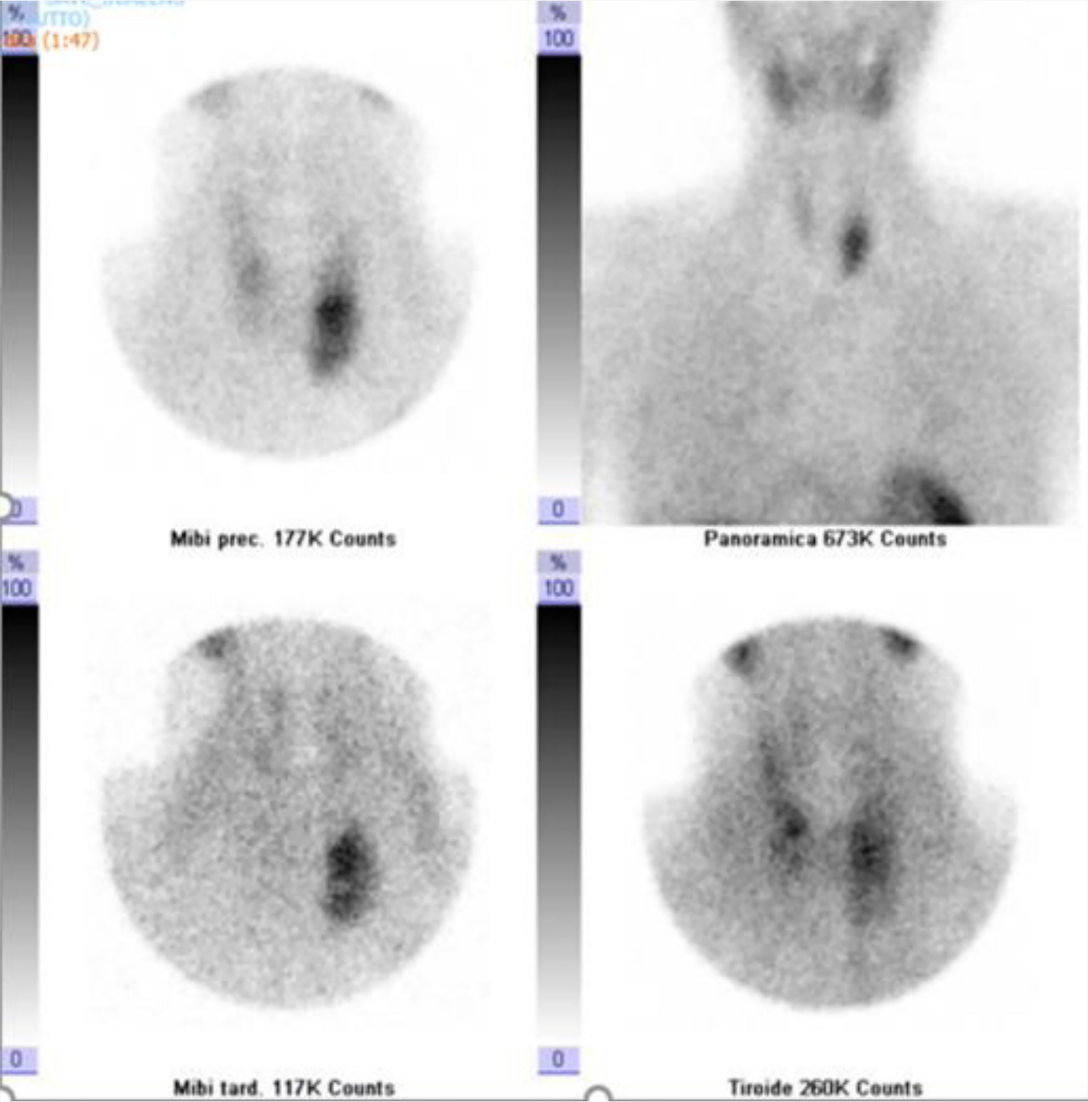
Dual-phase Tc-99m sestamibi scintigraphy. Early (5 and 30 min.) and delayed (60 and 120 min.) images showed a persistent accumulation of radiopharmaceuticals in the left inferior parathyroid region.
